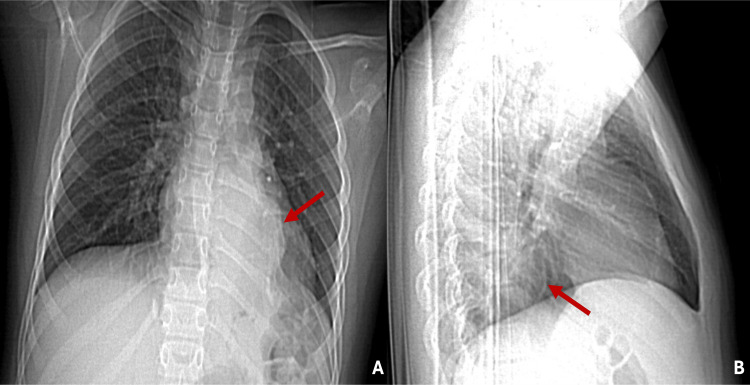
Chest X-ray in posteroanterior and lateral projections showing a retrocardiac intrapulmonary mass in the left lower lung (red arrows in A and B).
